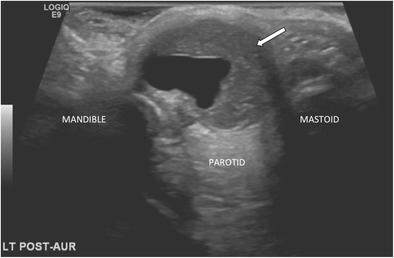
Transverse ultrasound (US) image from the same child as in Fig. 5, confirming the presence of a thick-walled cystic structure that was part of the sinus tract that extended to the left external auditory canal (*white arrow*)
